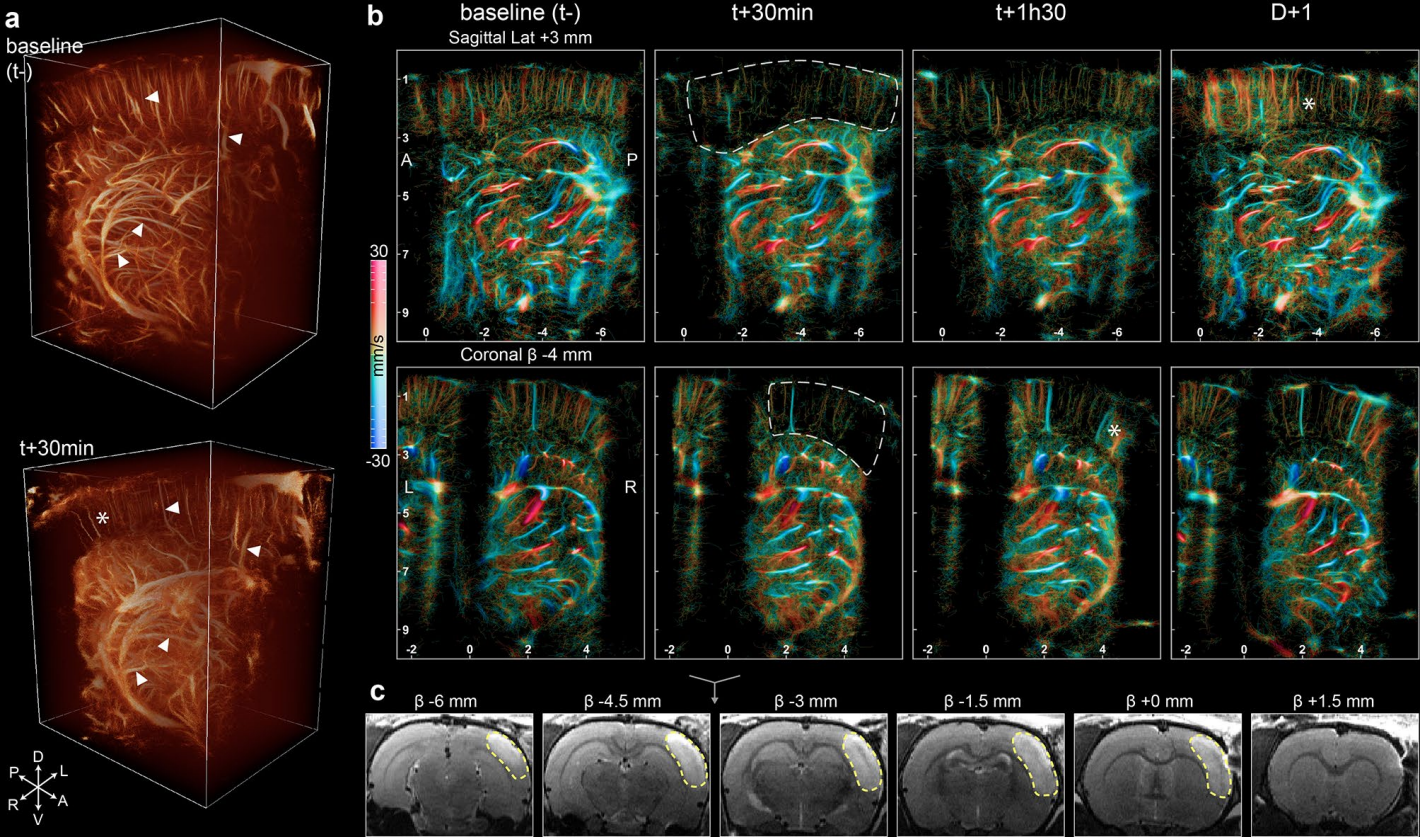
The MCA occlusion ischemic stroke was observed with 3DULM at the early phase. (a) 3D renderings at before and 30 min after stroke’s induction (Supplementary Video S1). (b) Sagittal and coronal slices of 600 µm with velocity rendering at different timepoints. Ischemic cortex wrapped with a white dotted line. $${V}_{max}=30\mathrm{ mm}/\mathrm{s}$$. Upward flows: red, downward flows: blue. (c) T2 MRI at D + 1 with the segmentation of the lesion (yellow dotted lines). Scale bar: 2 mm. Figures were created with (a) Amira 2019.4 (https://www.thermofisher.com/amira-avizo), (b,c) MatLab 2019a (https://www.mathworks.com/products/matlab).
